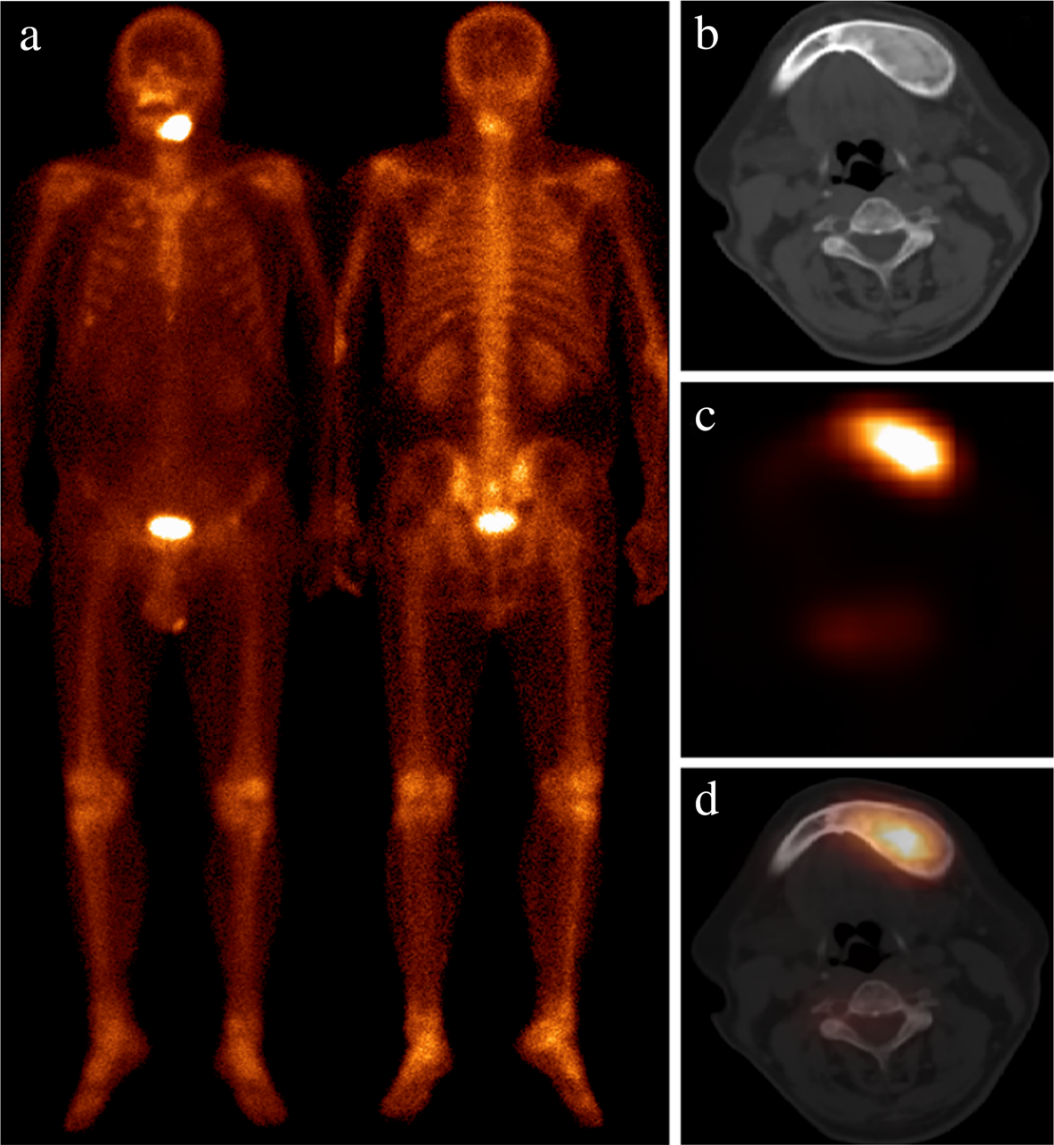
Patient 1 presented with newly diagnosed lung cancer. The WBS (a) demonstrated markedly increasing ^99m^Tc-MDP uptake in the left facial bone. Axial CT (b), SPECT (c), and hybrid SPECT/spiral CT imaging (d) demonstrated increasing ^99m^Tc-MDP uptake corresponding to expansion and ground glass density on mandible. A biopsy was subsequently performed and pathologic analysis confirmed the diagnosis of FD
